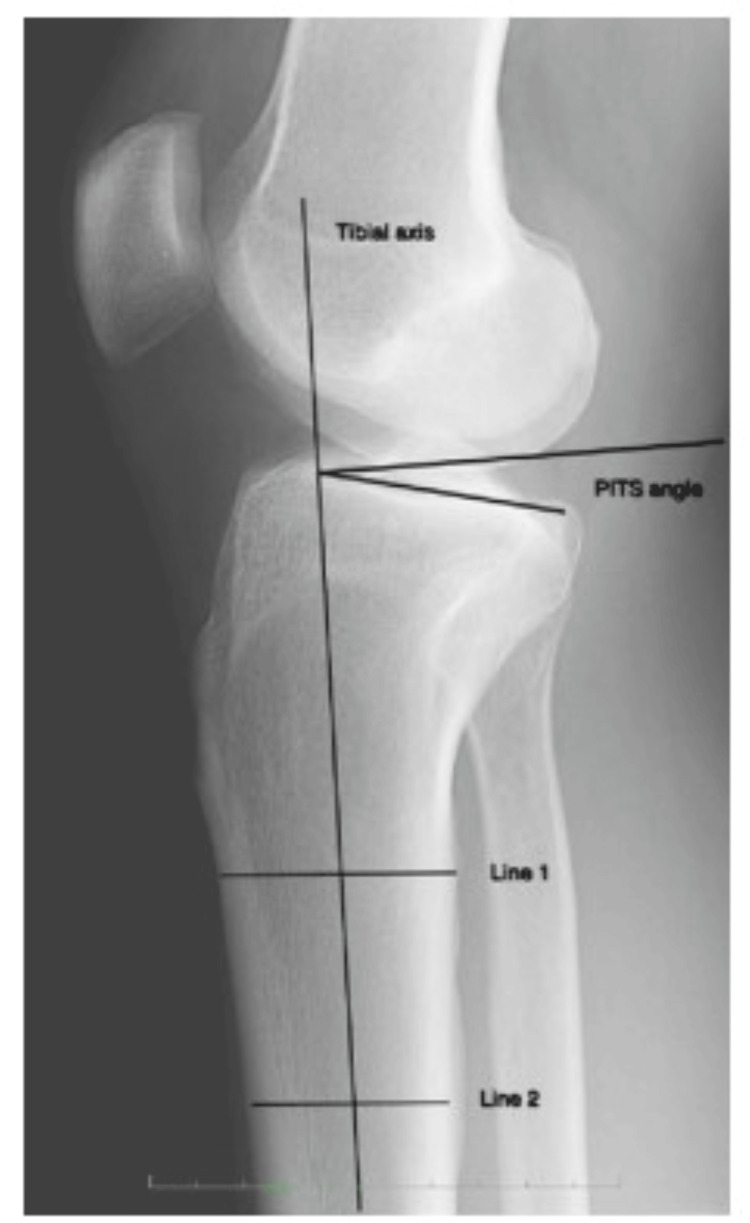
Tibial slope measurement on direct radiographs.
